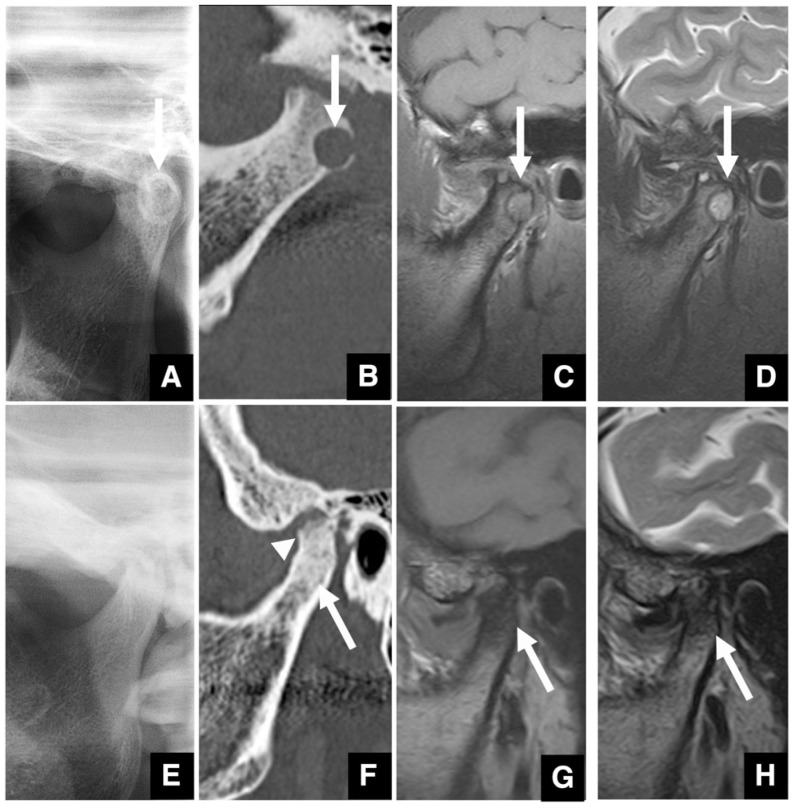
Imaging findings of osteoarthrosis of the temporomandibular joint. (A) Panoramic radiograph of a patient with a subchondral cyst and sclerotic changes (arrow) in the mandibular condyle. (B) MDCT corrected sagittal image (slice thickness, 2.0 mm) of the same patient as in (A) shows a subchondral cyst (arrow) and sclerotic changes in the mandibular condyle. MRI T1-weighted image (TR 1050 ms, TE 18 ms, slice thickness 3.0 mm) of the same patient as in (A). (C) The signal intensity of the subchondral cyst (arrow) in the mandibular condyle is higher than that of the muscle. MRI T2-weighted image (TR4000 ms; TE 108 ms; slice thickness 3.0 mm) of the same patient as in (A). (D) The subchondral cyst (arrow) in the mandibular condyle shows high signal intensity. (E) Panoramic radiograph and (F) MDCT corrected sagittal image of a patient with sclerotic changes (arrow) in the mandibular condyle, glenoid fossa, and articular eminence and erosive changes (arrowhead) in the mandibular condyle. (G) MRI T1-weighted image and (H) MRI T2-weighted image of the same patient as in (E). The mandibular condyle shows no signal (arrow) throughout. Permission has been obtained to publish all figures.
